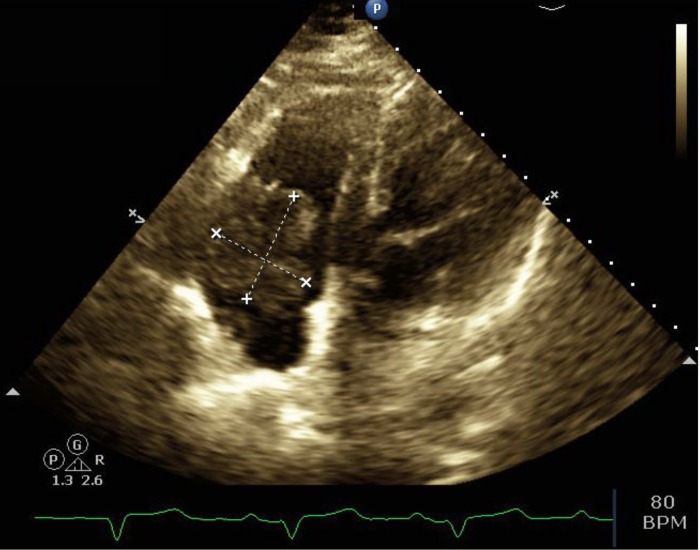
Four chamber echocardiogram demonstrating a large, mobile echodensity measuring 4.39cm x 4.12cm in the tricuspid valve and anterior atrioventricular wall, extending into the right atrium and ventricle.
